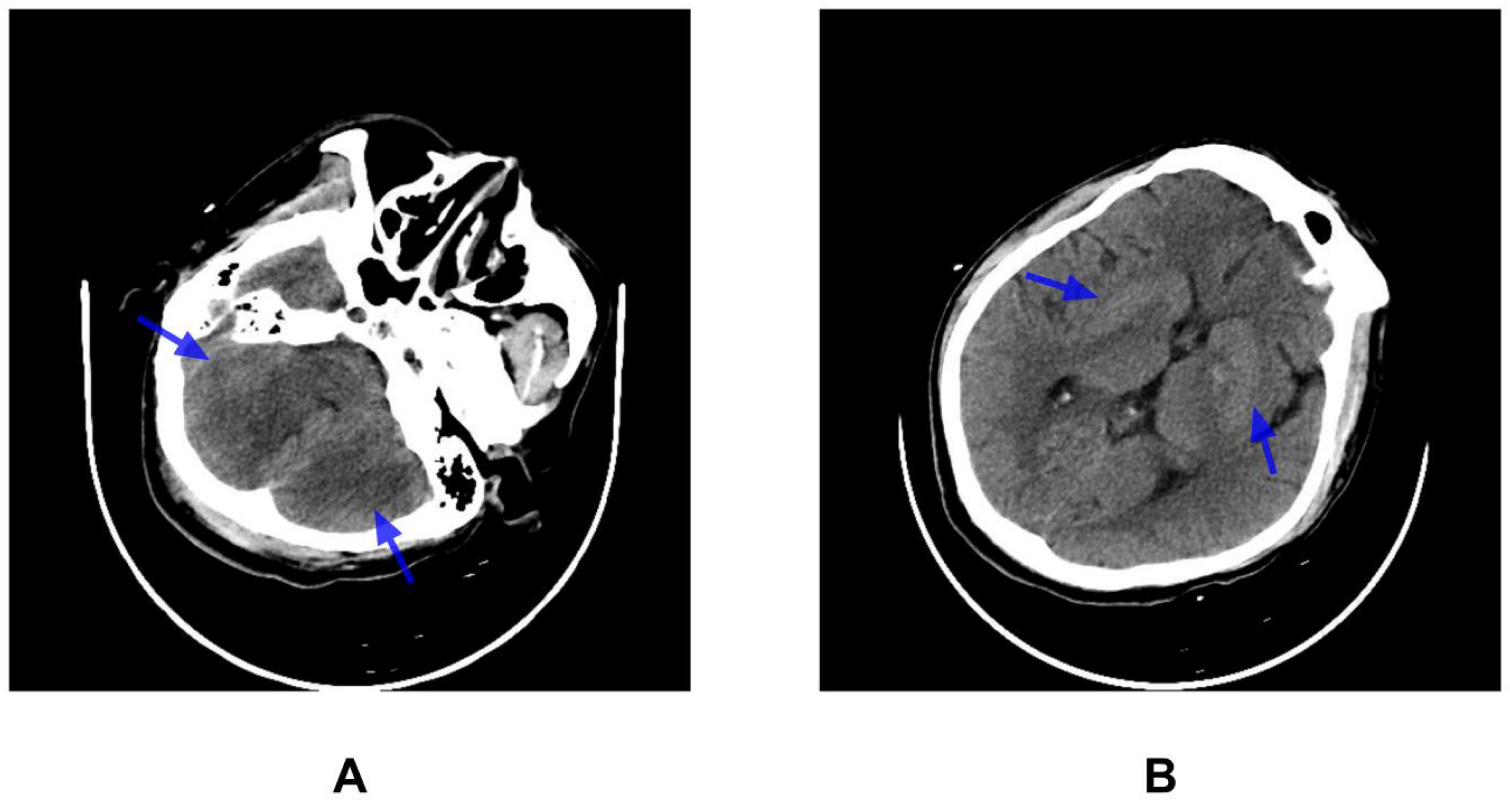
Brain CT findings (day 9). CT scan showing (A) bilateral hypodense lesions in the cerebellar hemispheres and (B) slightly hyperdense mixed-density lesions in the bilateral basal ganglia, as indicated by the blue arrows. These findings are largely unchanged compared with the CT performed on July 19, 2022.
